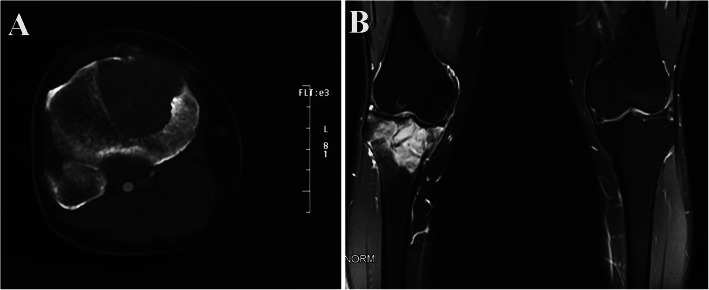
CT scan (a) and MRI (b) of right tibia showed a lytic lesion in the proximal tibia without presence of soft-tissue mass
